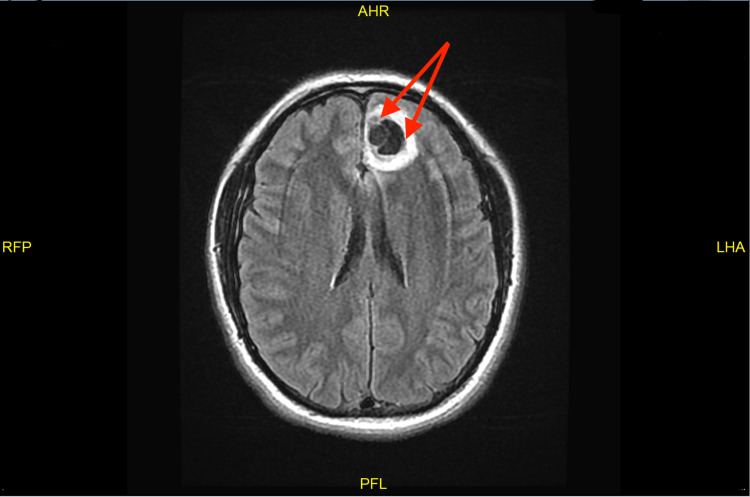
MRI head showing a bilobed cystic mass in the anteromedial left frontal lobe MRI: magnetic resonance imaging
